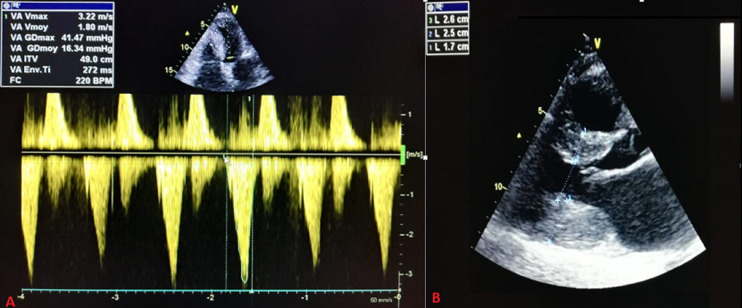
transthoracic echocardiography showing: A) a max instantaneous late peak gradient of 42 mmHg measured with continuous wave (CW) Doppler; B) a posterior wall thickness at 26 mm and a septum thickness at 17 mm measured on a parasternal long axis view
